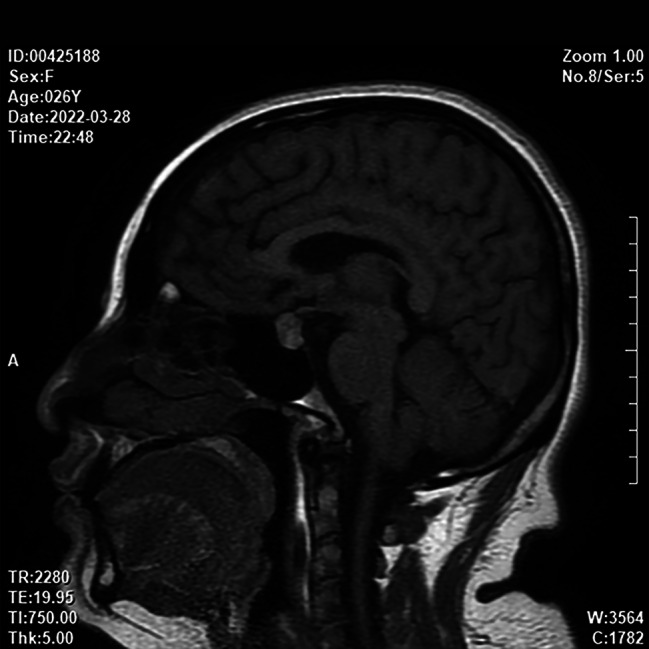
MRI of the brain showed that the pituitary gland was plump
